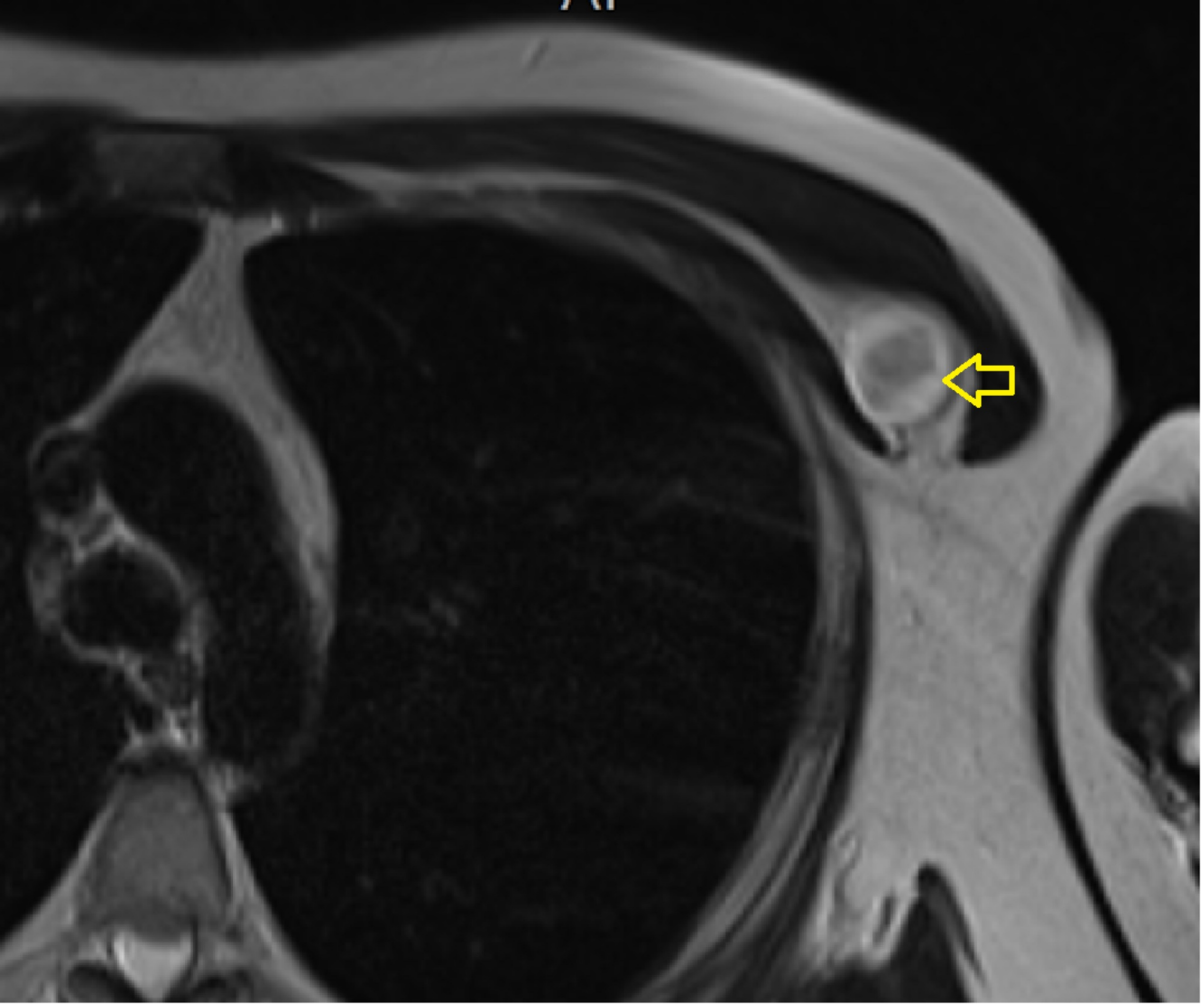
T2-weighted MRI of the chest Heterogeneously hyperintense, well-defined lesion (arrow) within the intermuscular plane, intervening between the left-sided pectoralis major and pectoralis minor muscles. MRI: magnetic resonance imaging
